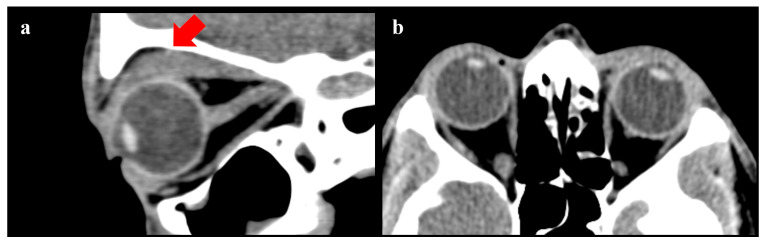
CT scan performed 5 months after surgery. (a) Sagittal view—the LM/SR muscle complex increase is highlighted in red. (b) Coronal view.
